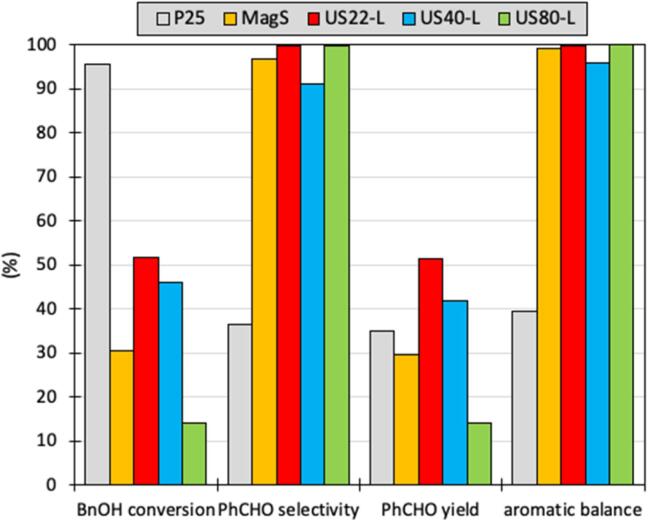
Photocatalytic performance of the photocatalytic oxidation of benzyl alcohol to benzaldehyde for the samples synthesized under ultrasound irradiation of different frequency (22, 40 and 80 kHz), in silent using magnetic stirrer and the commercial TiO_2_ P25, under UV light exposure for 6 h.
